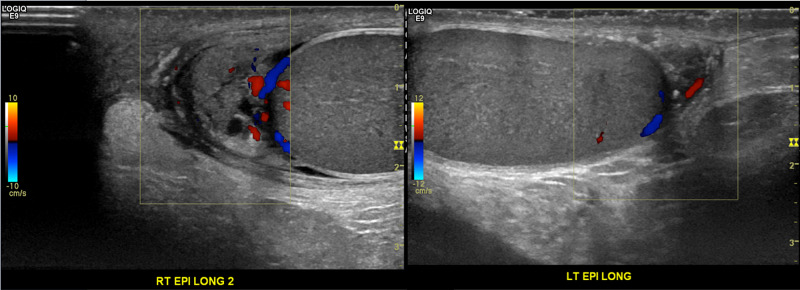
Doppler ultrasonography of the bilateral epididymides Doppler ultrasonography revealed normal vascularity across the bilateral epididymides.
